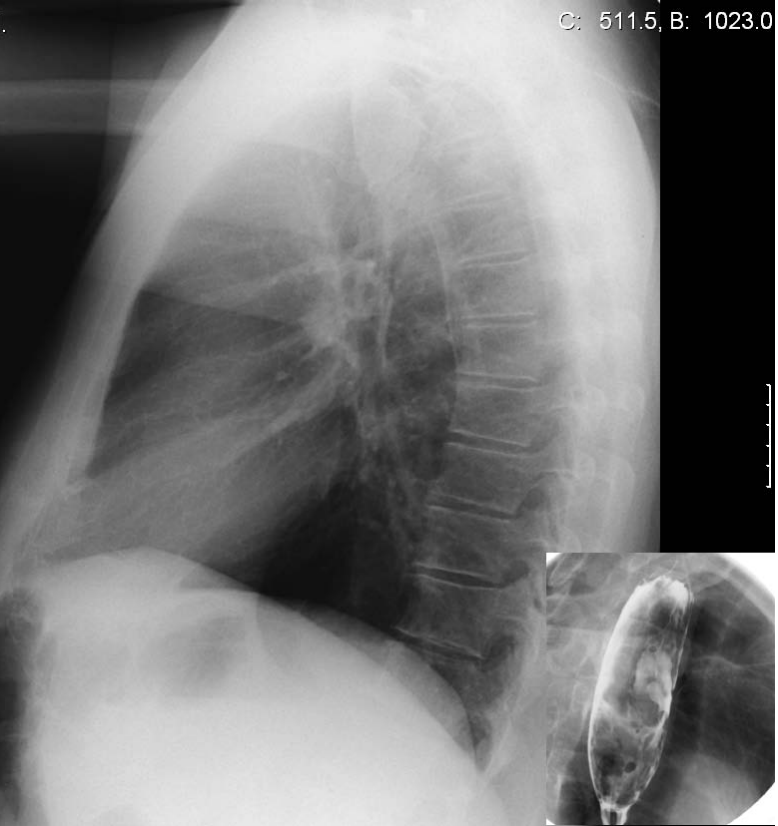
Lateral chest x-ray showing a shadow ventrally of the vertebra in the posterior mediastinum. Insertion shows a barium swallow with a large tubular intraluminal mass in the proximal esophagus.
